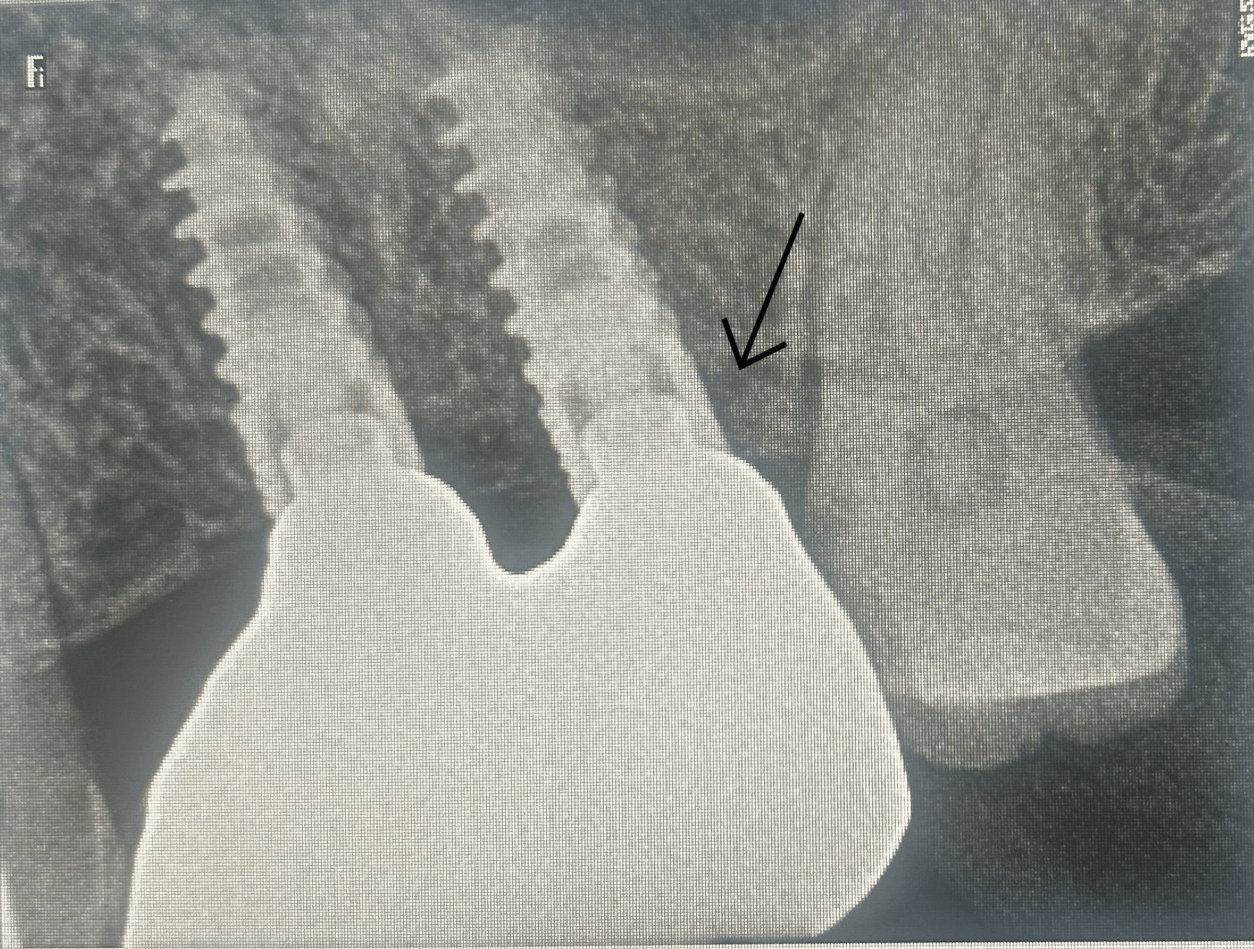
Follow-up IOPA after six months of prosthetic loading. The arrow indicates the marginal bone stability. IOPA: intraoral periapical radiograph
